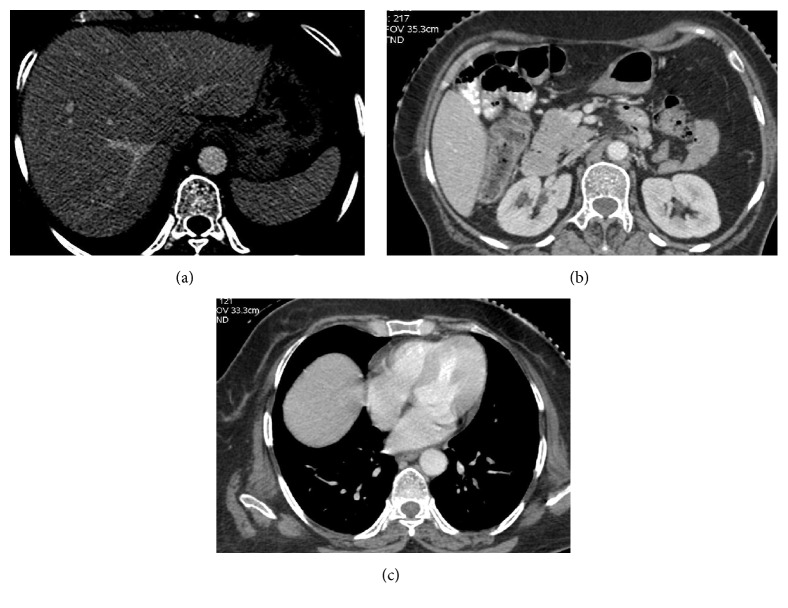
CT scan after six cycles of chemotherapy reveals complete resolution of the liver, pancreatic, and chest wall lesions.
